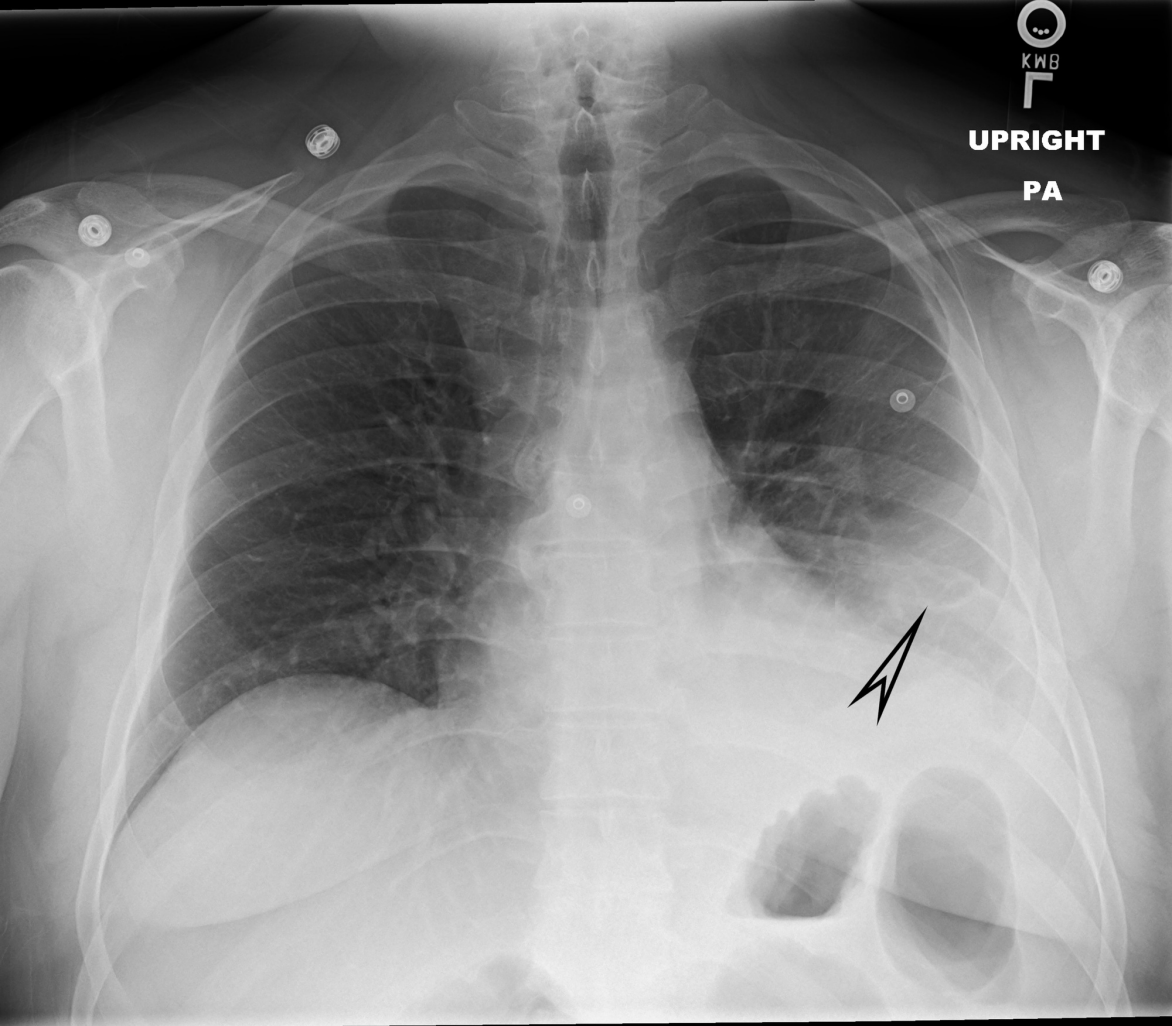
Chest X-ray highlighting a patchy infiltrate in the left lower lobe with a left pleural fusion and no pneumothorax (arrowhead)
